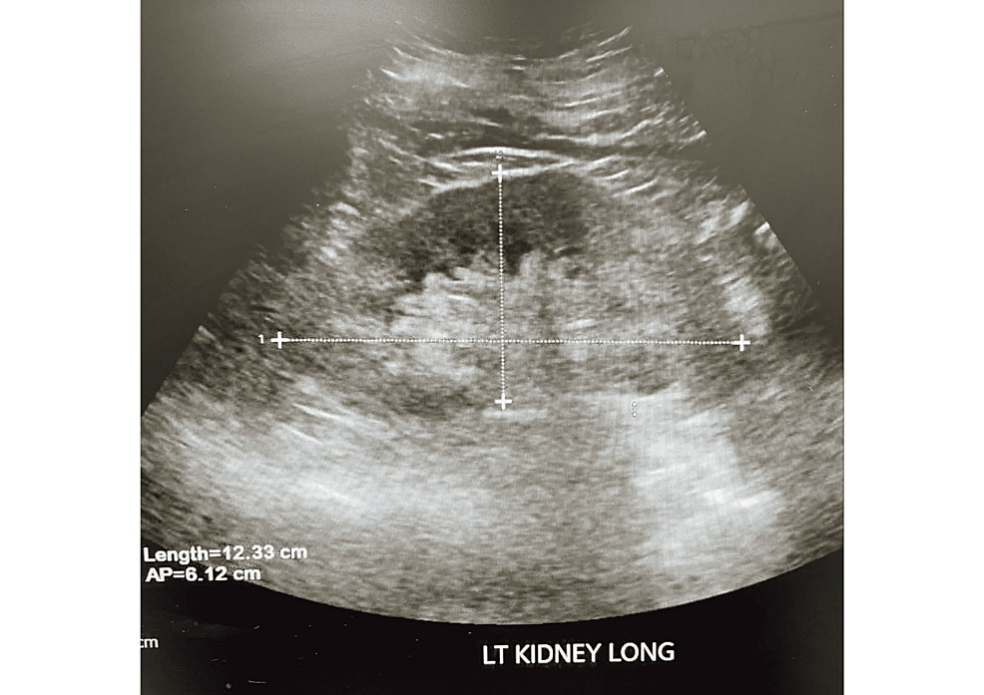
Ultrasound of left kidney showing diffuse increased echogenicity of renal parenchyma consistent with chronic renal disease.
